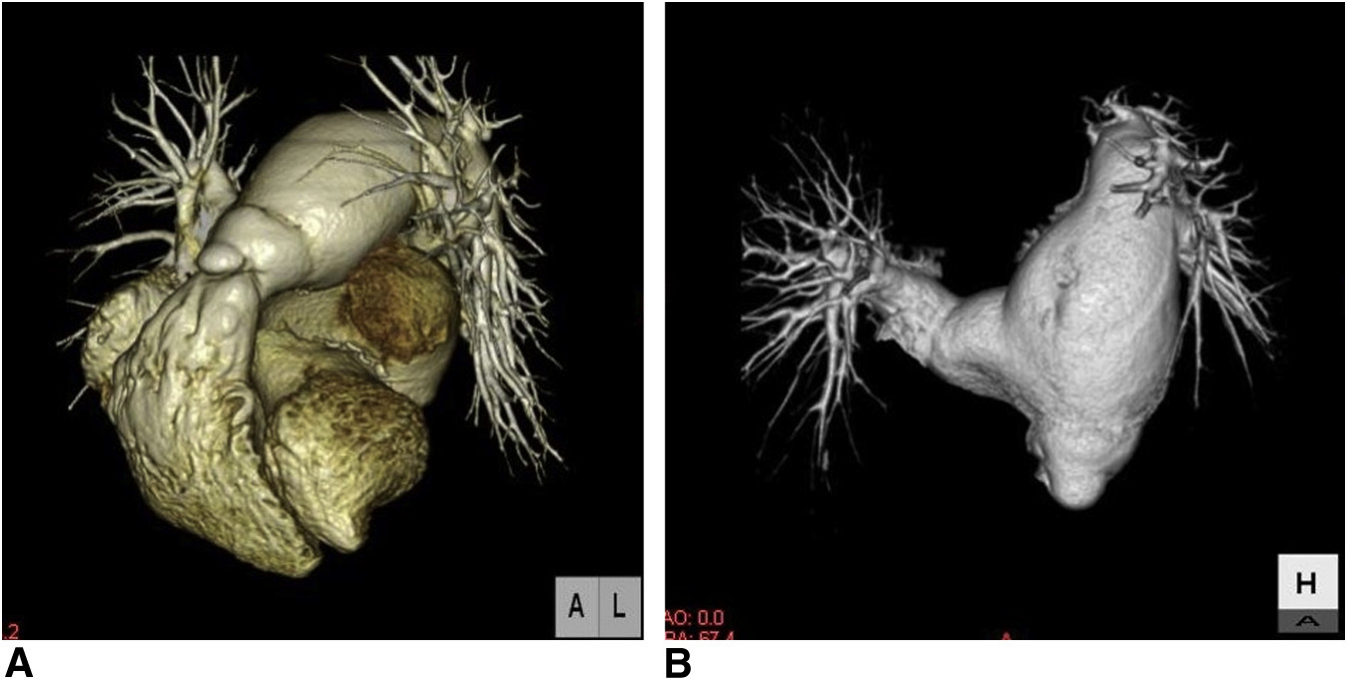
A and B, Postoperative computed tomography. A reduction is indicated in diameter of the main pulmonary artery to 52 mm, from the preoperative value of 66 mm.
